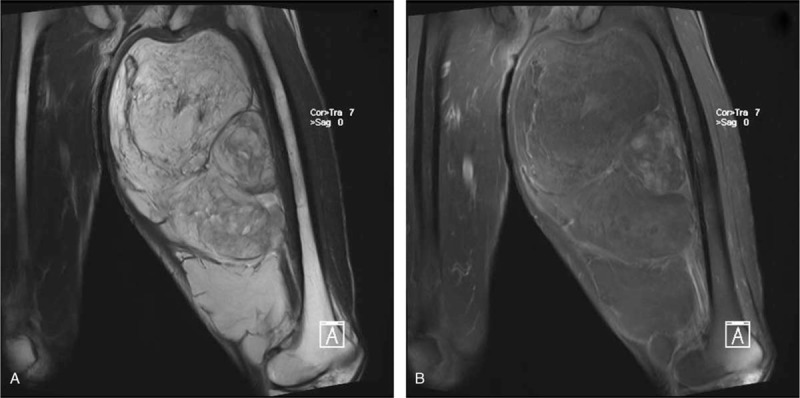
Preoperative magnetic resonance imaging findings. There was a huge agglomerate short T1 (A) and long T2 (B) signal in the left thigh muscle space, which was about 30 × 20 × 11 cm.
